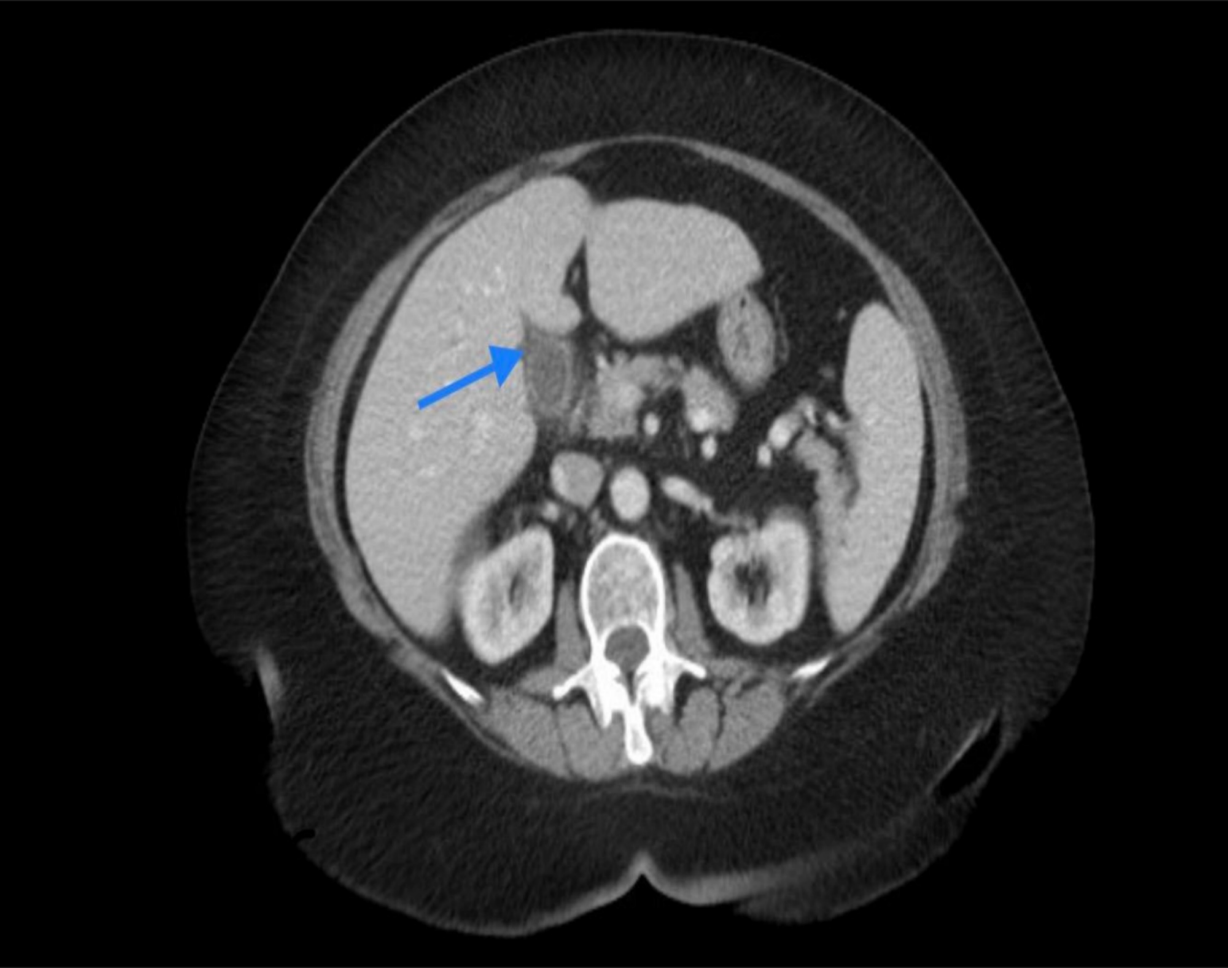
CT scan of the abdomen Acute emphysematous cholecystitis (blue arrow)
